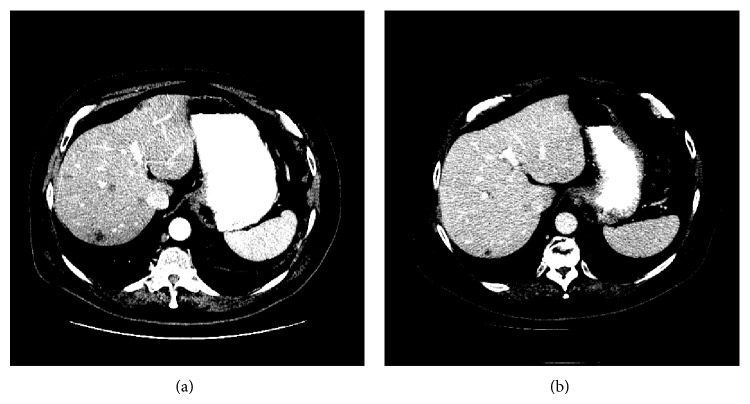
CT of the abdomen in case #3 revealed liver metastases (a) before the treatment and (b) after 17 months of treatment.
